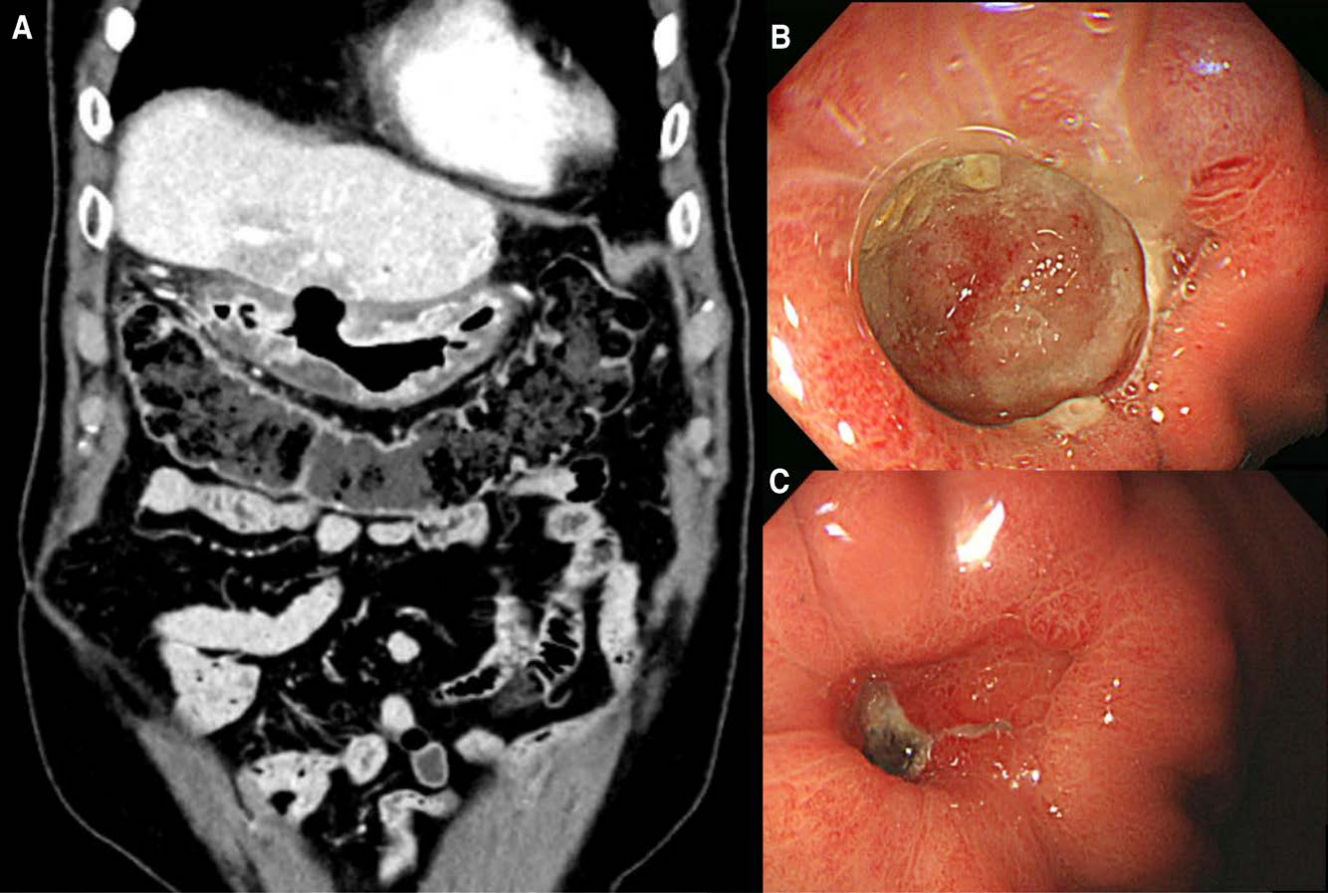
CT and gastroendoscopy image. (A) A deep ulcer in the stomach in the CT (coronal view). (B) A deep ulcerative lesion in the gastroendoscopy. (C) Follow-up gastroenteroscopy image. CT = computed tomography.
